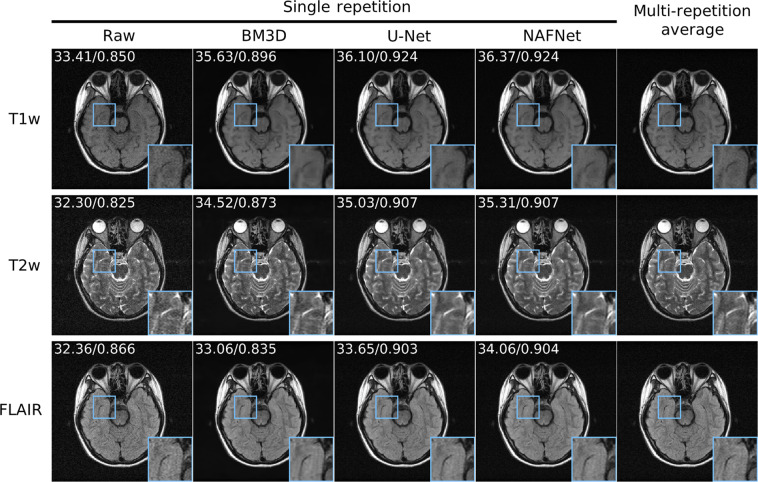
Results of the pilot M4Raw dataset demonstration for data-driven MRI denoising. The mean PSNR and SSIM values were calculated for each contrast on the validation subset and labeled on top of the representative images. Both data-driven methods achieved higher PSNR and SSIM values than the traditional BM3D method, offering visually improved images with less blurring.
